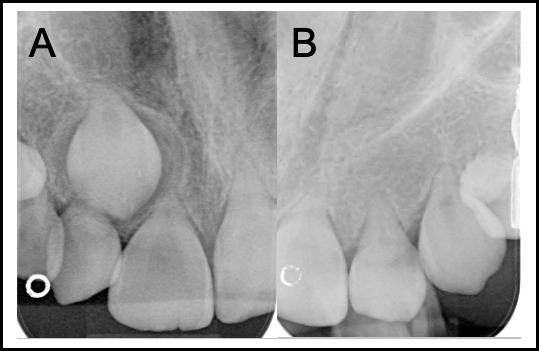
Anterior periapical radiographs taken 8.5 years after treatment showing the short tapering root form of the UR3, UR2, UR1, UL1, UL2, and UL3. (A) Periapical radiograph maxillary right anterior segment. (B) Periapical radiograph maxillary left anterior segment.
